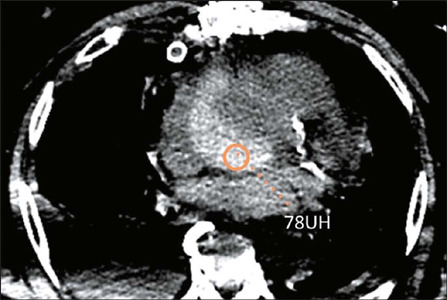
Mediastinal hematoma. Axial CT image (mediastinal window) showing a
